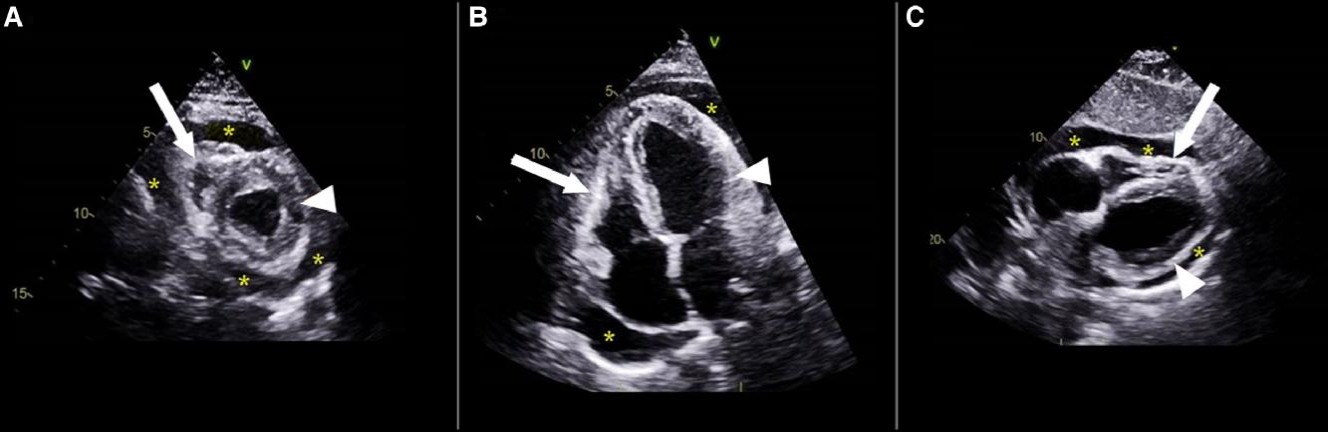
Echocardiographic imaging in 3 essential views. The views include the parasternal short-axis (A), apical 4-chamber (B), and subcostal (C). Pericardial effusion (asterisks) can be seen encompassing all chambers of the heart with a collapse of the right ventricular free wall (white arrow). The left ventricle is normal (white arrowhead).
